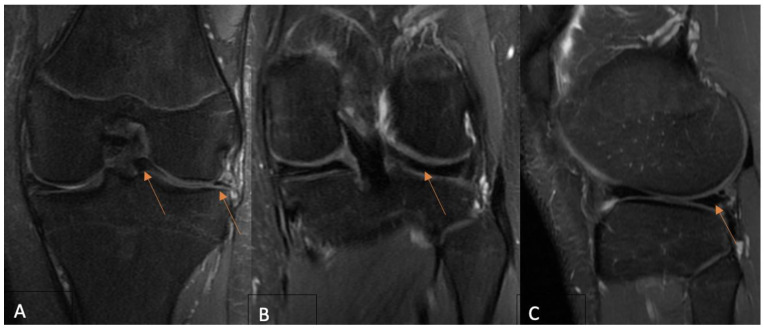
(A) A preoperative coronal PD-FSE, FS MR image shows (arrows) a bucket-handle-type lateral meniscus tear in a 16-year-old boy. Twelve-month postoperative coronal (B) and sagittal (C) PD-FSE, FS MR images reveal (arrows) a repaired lateral meniscus and no intrameniscal signal alterations—MRI grade 1 due to Crues and Stroller classification. MR—magnetic resonance; FSE—fast spin echo; FS—fat saturation.the figures (MRI views) indicate the meniscus structures in the knee joint and the tears (arrows) within the meniscus tissue.
